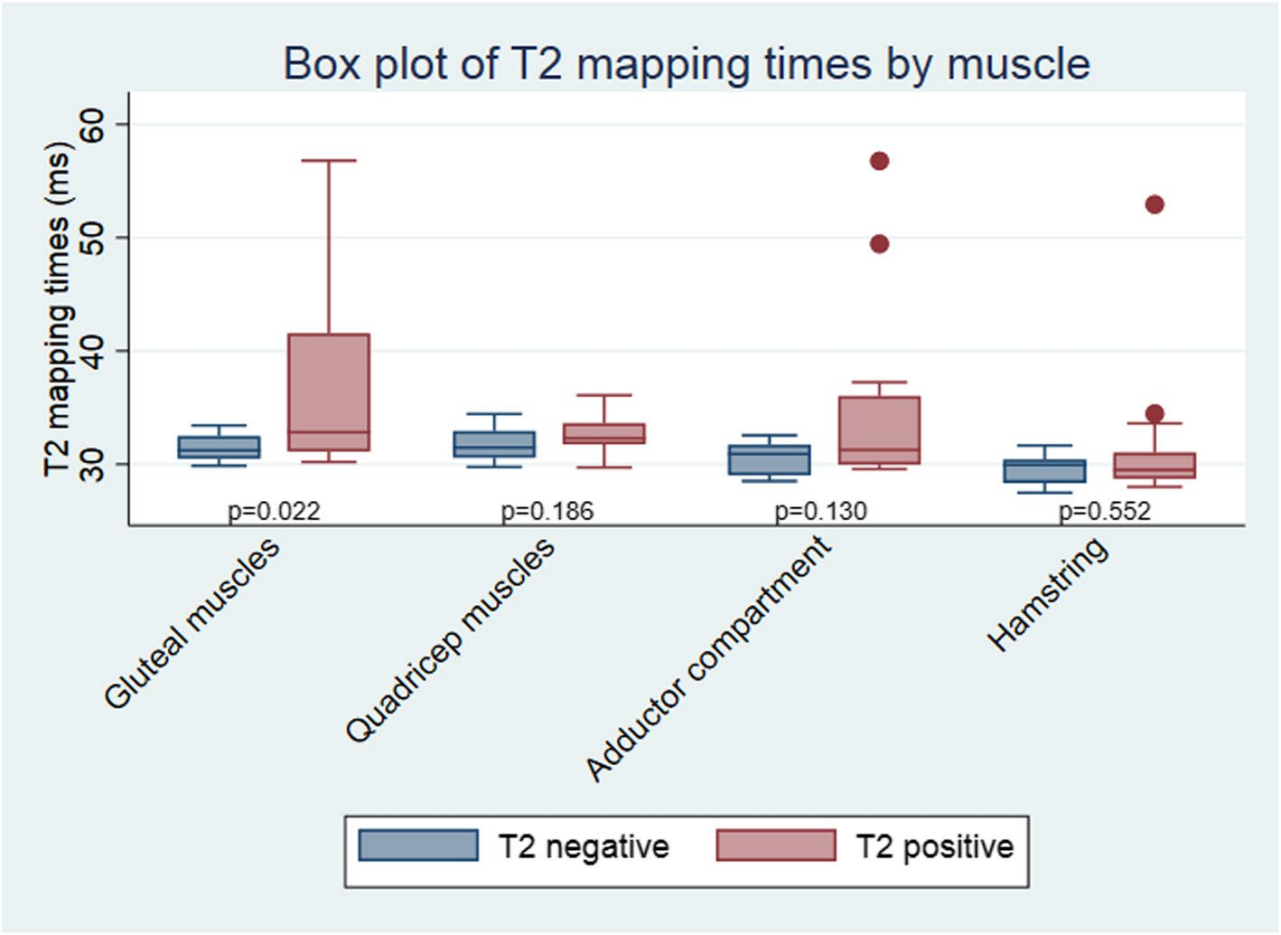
Comparison of T2-mapping times to radiologist-assessed muscle oedema. T2 negative: normal T2 signal on MRI indicating no oedema; T2 positive: T2 hyperintensity recorded indicating muscle oedema
